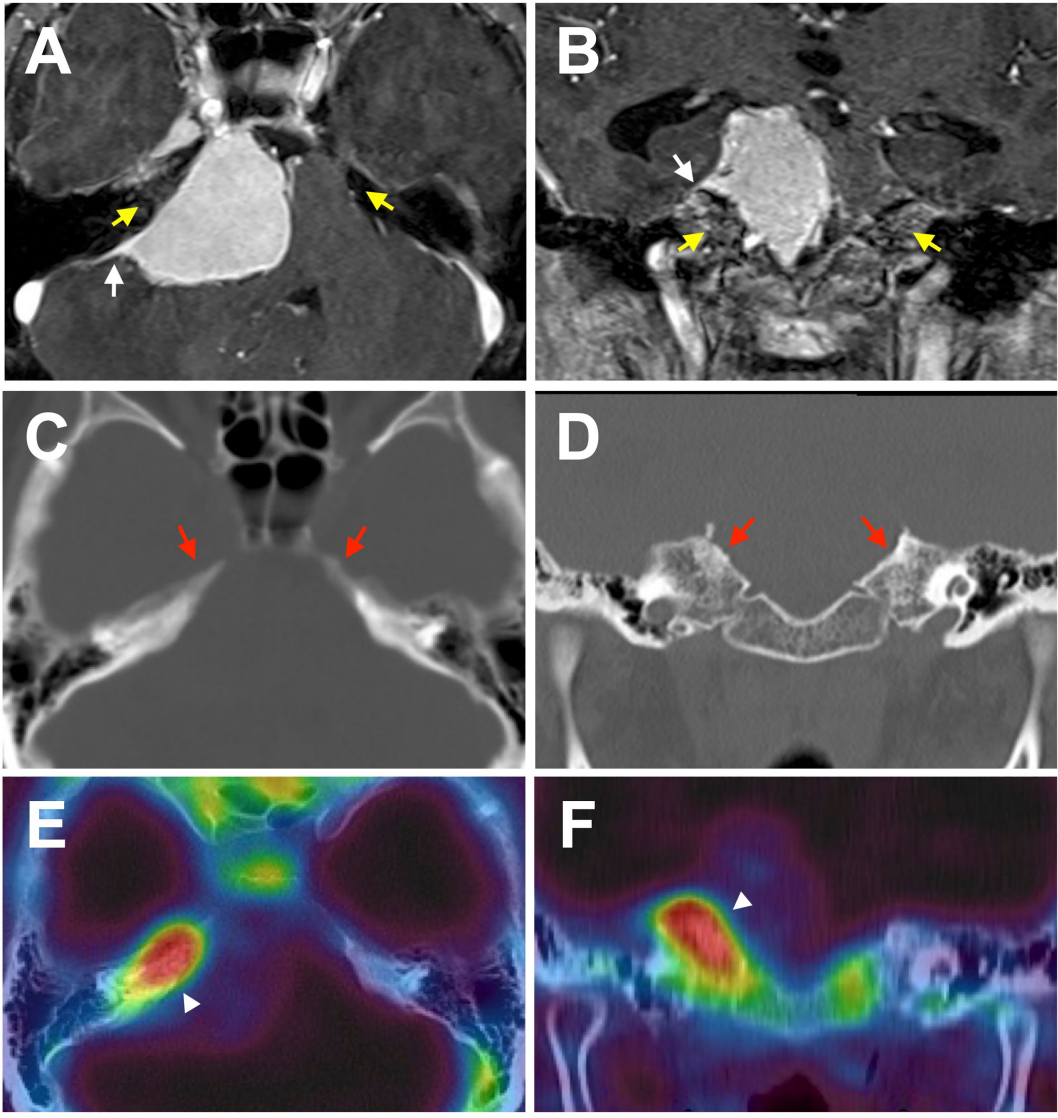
A representative case of meningioma with bone invasion. Axial (A, C, E) and coronal (B, D, F) images demonstrating a petroclival meningioma. (A, B) T1-post contrast MRI shows a characteristic dural tail (white arrows). Enhancement in the adjacent bone is ambiguous, and no obvious laterality is found (yellow arrows). (C, D) Non-contrast bone CT does not reveal a hyperostosis with tumor-associated laterality. (E, F) However, F^18^ fluoride PET/CT fusion image indicates prominent uptake in the adjacent bone suggesting bone invasion of the tumor (white arrowheads).
